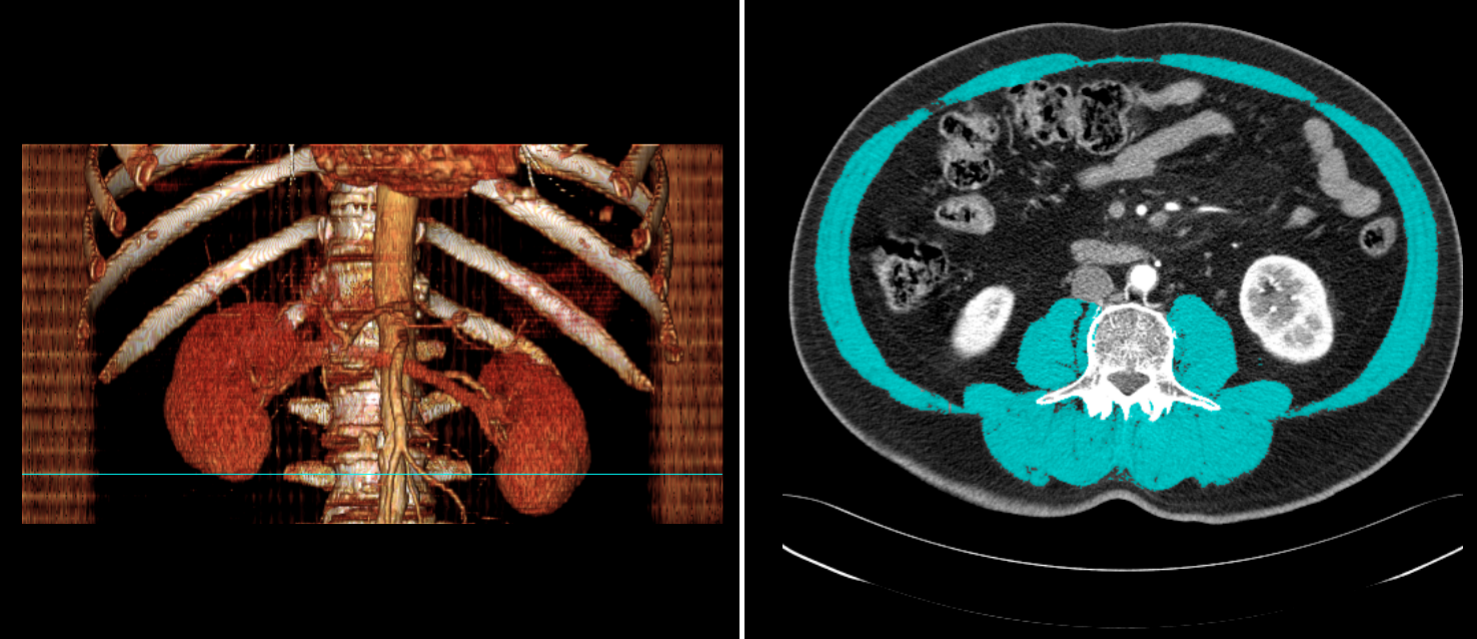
Computed tomography images of the region of the third lumbar vertebra, with skeletal muscle highlighted in blue (-29 to 150 Hounsfield units). The blue line on the three-dimensionally reconstructed image on the left indicates the level of the third lumbar vertebra, which is shown in the axial view on the right.
